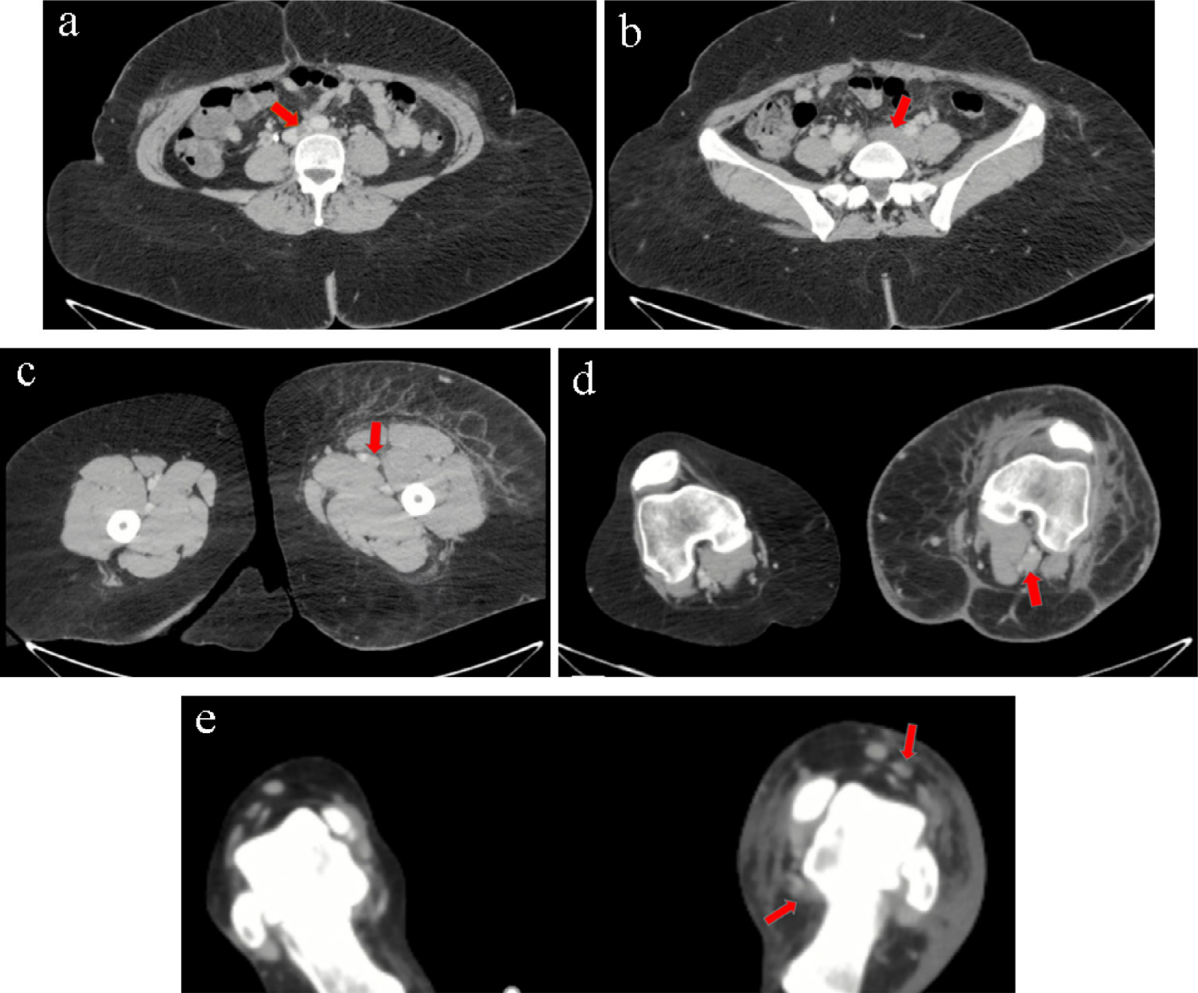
Lower extremities CT angiography shows no flow with extensive thrombus fills the lumen of the distal IVC (A), left common iliac vein (B), left superficial femoral vein (C), left popliteal vein (D), left distal posterior tibial vein and distal anterior tibial vein (E). Also note that there is no contrast filled the lumen of the vein, indicating total occlusion. IVC, inferior vena cava.
